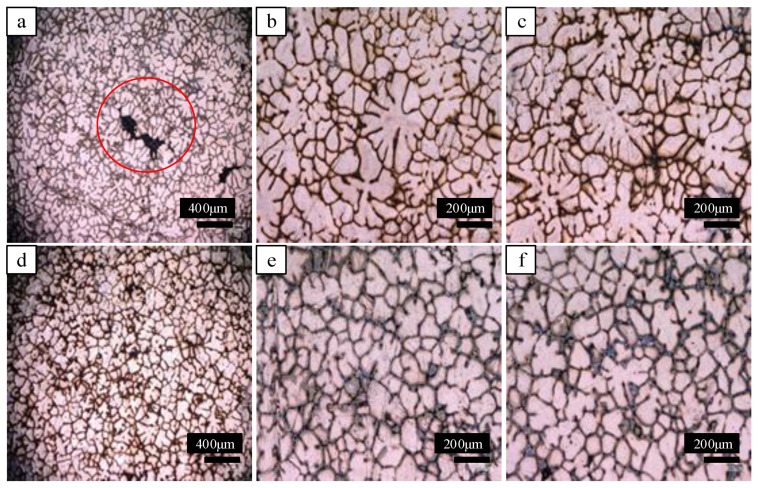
Grain structure of conventional and ultrasonic ingots: (a) edge of conventional ingot; (b) 1/2 radius of conventional ingot; (c) center of conventional ingot; (d) edge of ultrasonic ingot; (e) 1/2 radius of ultrasonic ingot; (f) center of ultrasonic ingot.
